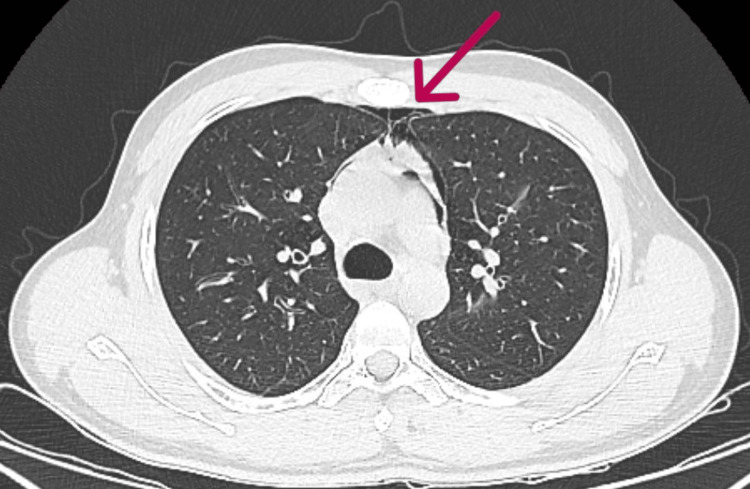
Repeat chest CT chest showing reduced air pockets around the great vessels and in the pericardium (taken July 26, 2022).
